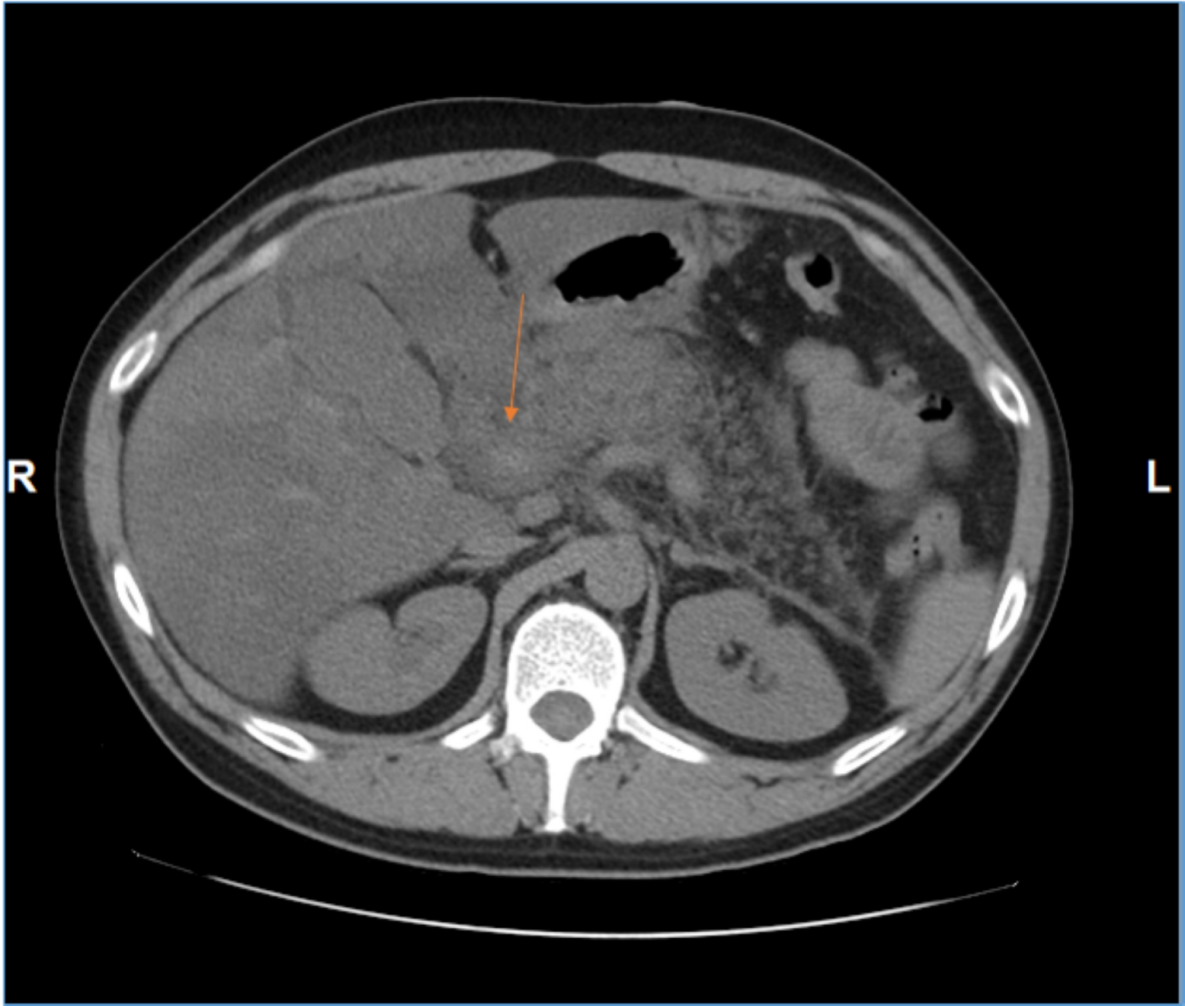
CT scan abdomen. CT scan abdomen shows that the pancreatic head is enlarged (the arrow), ill-defined and surrounded by fluid and fat stranding, consistent with acute pancreatitis. Inflammatory fluid and fat stranding throughout most of the upper abdomen. No walled fluid collections to indicate pancreatic pseudocyst or abscess.
